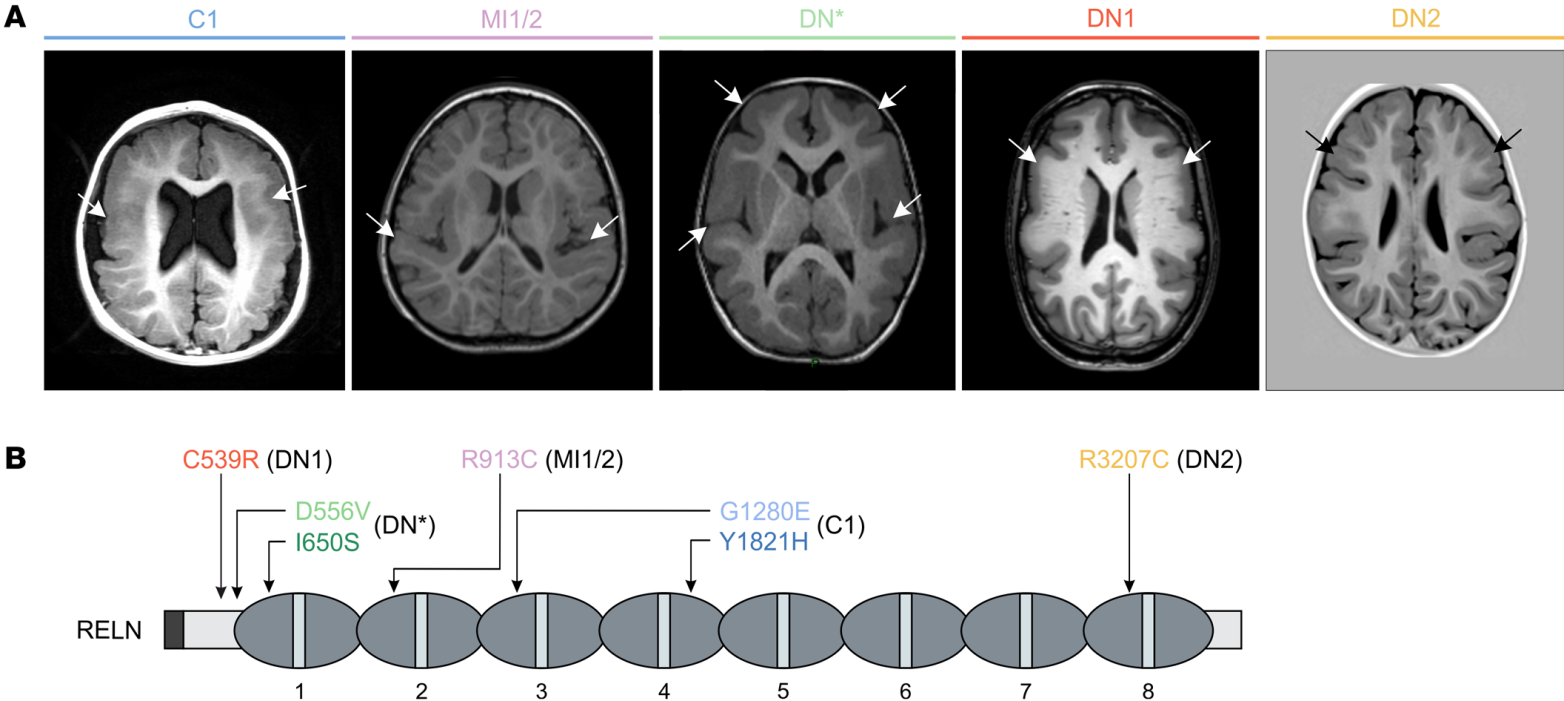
Cortical malformations in heterozygous patients associated with *RELN* missense variants. (A) Brain MRI from patients with heterozygous *RELN* variants. C1 exhibits bilateral fronto-parietal polymicrogyria with nodular heterotopia, MI1/2 bilateral perisylvian polymicrogyria, DN* frontal-predominant bilateral pachygyria, and DN1 and DN2 frontotemporal-predominant bilateral pachygyria. Representative axial T1 section of the cortical malformations (white arrows). (B) Primary structure of the RELN protein showing 8 Reelin repeats (1–8 ovals). Arrows indicate the position of missense variants; each color corresponds to a patient (C1 blue, MI1/2 pink, DN* green, DN1 orange, and DN2 yellow).
